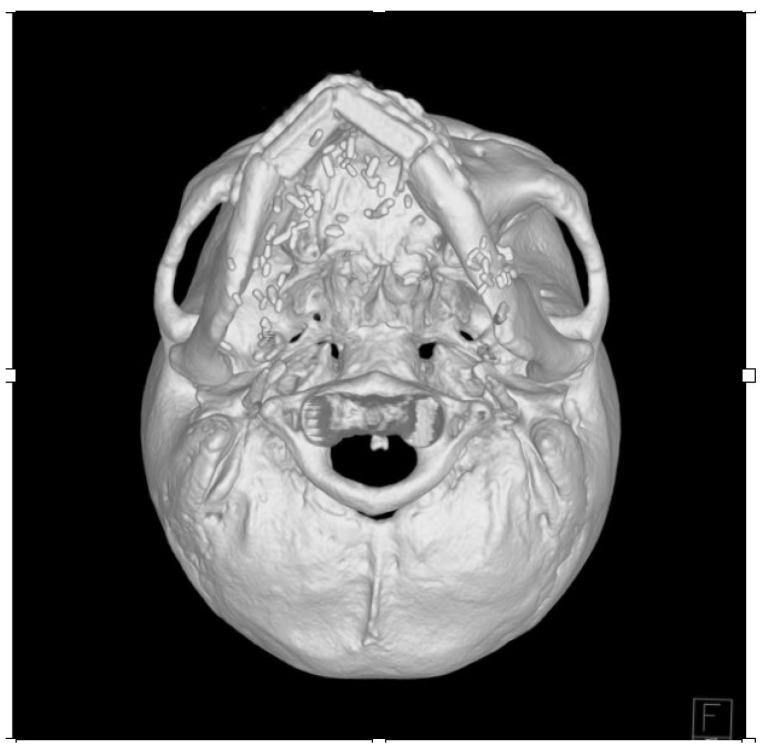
Postoperative computed tomography scan of achieving smooth mandibular contour.
